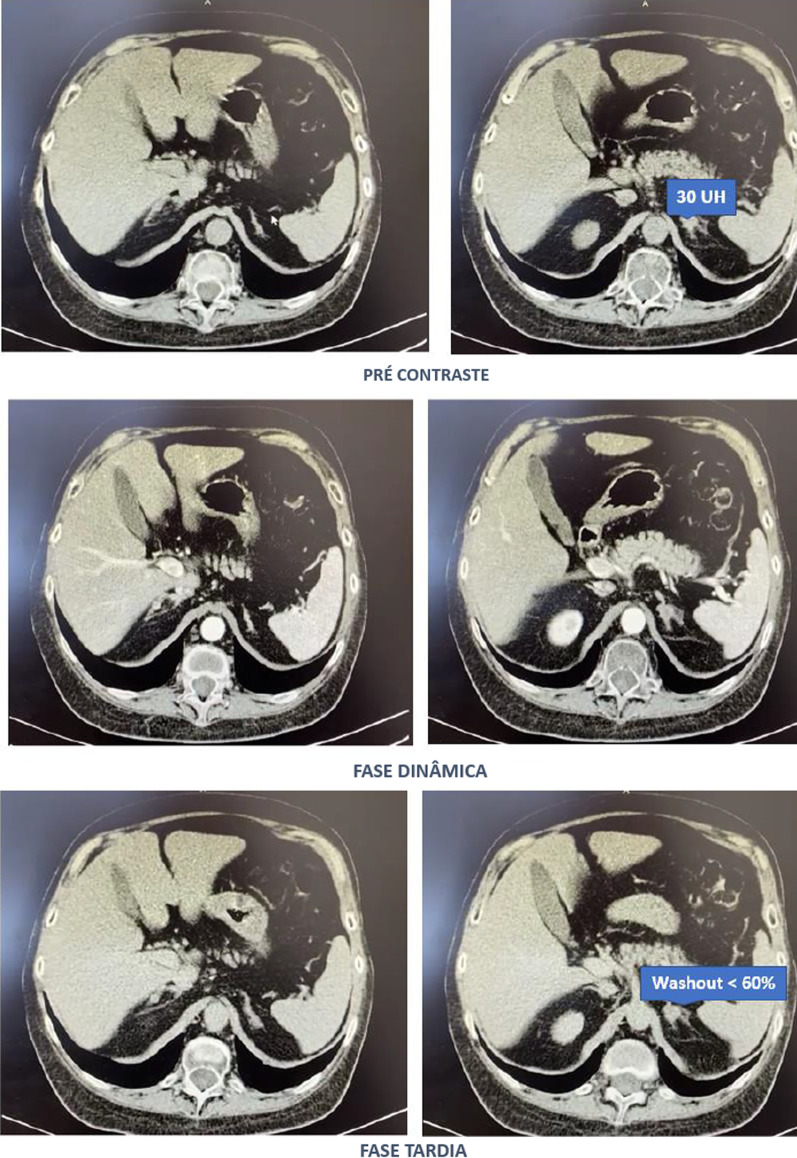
Computed tomography abdomen with adrenal protocol
